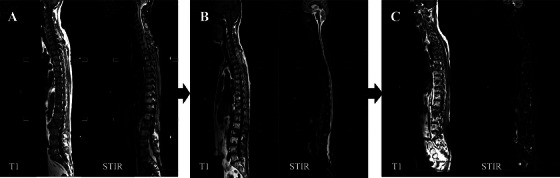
MRI findings of the whole spine, taken 56 days before (A), 26 days after (B), and 140 days after CAR‐T infusion (C), respectively. STIR, short inversion time inversion recovery; T1, T1‐weighted image.
